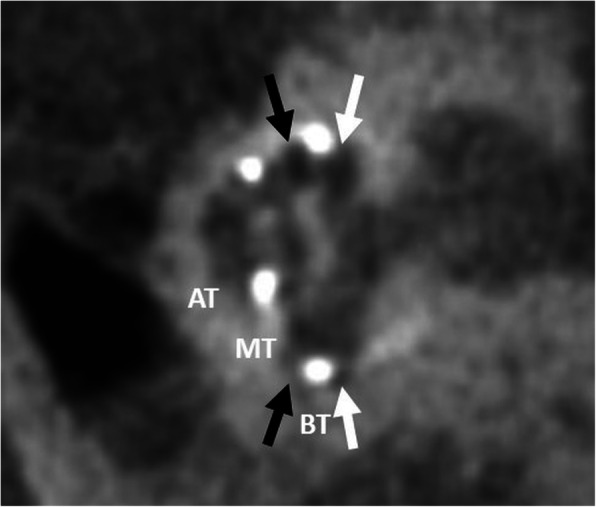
A 72-year-old male patient, with electrode lifting the basilar membrane. CBCT paracoronal image shows the electrode array located in a lateralized and elevated intermediate position between scala vestibuli (black arrow) and scala tympani (white arrow). BT – basal turn. MT – middle turn. AT – apical turn
